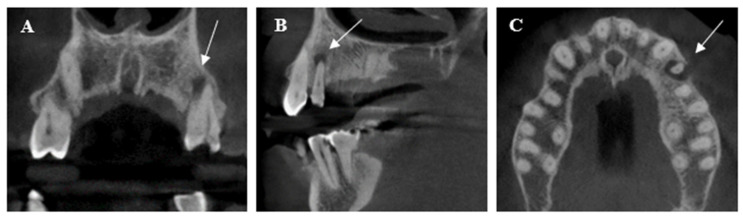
CBCT images showing periapical radiolucency of maxillary left second premolar (white arrow): (A) coronal, (B) sagittal, and (C) axial views.
